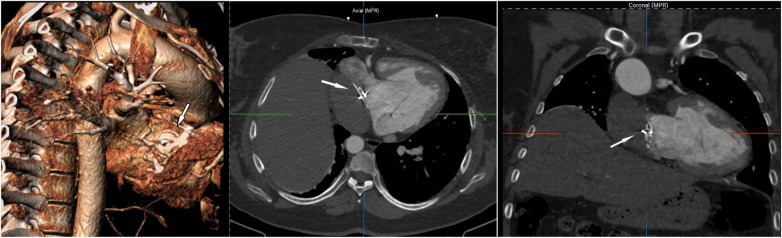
3D computed tomography assessment for optimal transbaffle puncture site selection relative to the occluder (arrows indicating the occluder).
